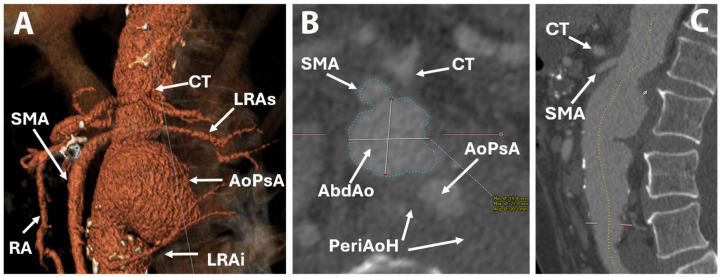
Computer tomography depicting origin of renal arteries: reconstruction (A), transverse view (B), and sagittal view (C). AoPsA—aortic pseudoaneurysm; CT—celiak trunck; LRAi—left renal artery inferior branch; LRAs—left renal artery superior branch; RA—Riolan arcade; SMA—superior mesenteric artery, AbdAo—Abdominal Aorta; PeriAoH—periaortic hematoma.
